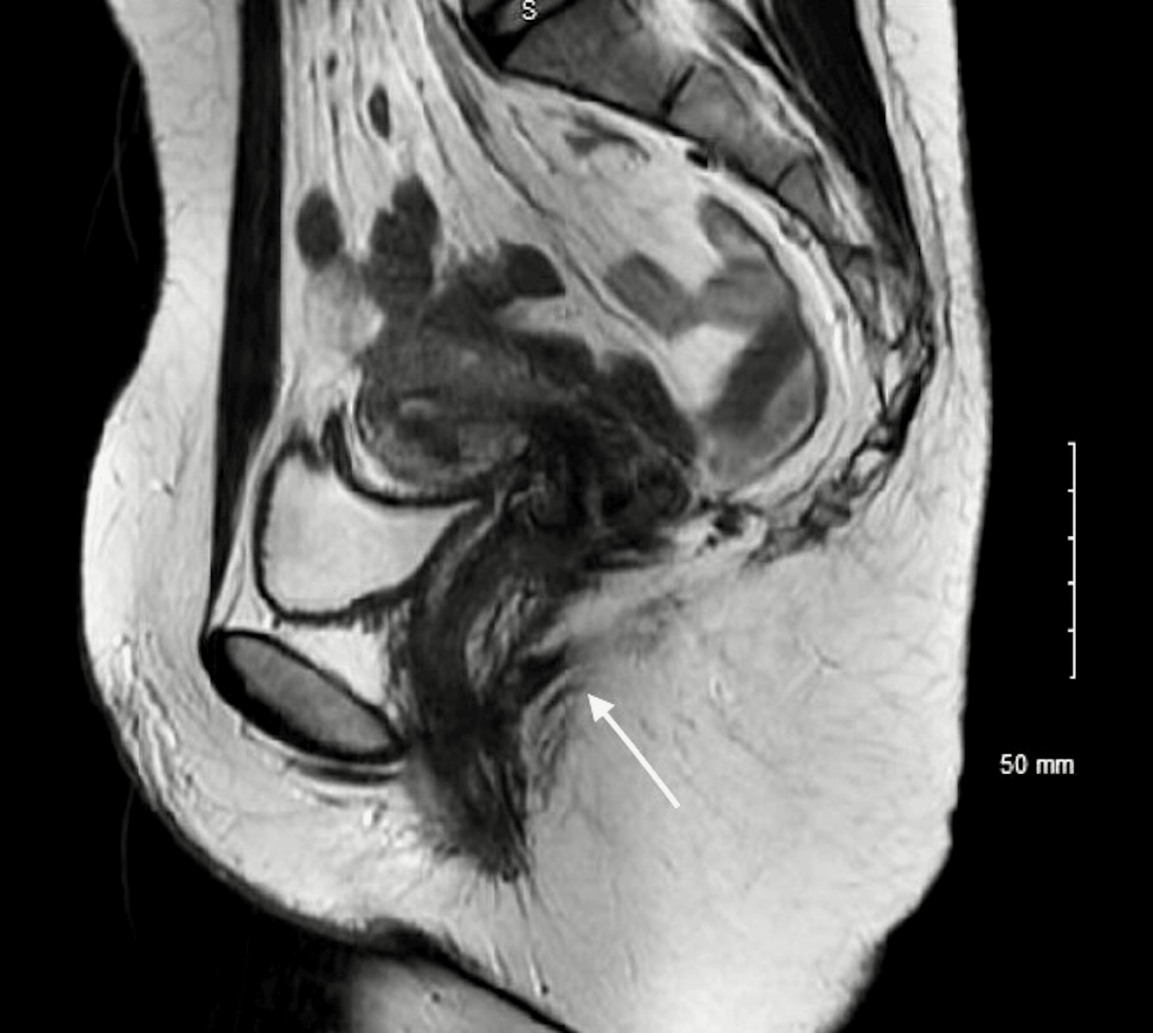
Pelvic MRI post-panproctocolectomy. Sagittal T2-weighted pelvic MRI demonstrates an enterovaginal fistula extending to the posterior vaginal vault. It was hypothesised that the fistula facilitates bacterial translocation into the vagina, with potential spread to the uterus, contributing to chronic endometritis. MRI: magnetic resonance imaging.
